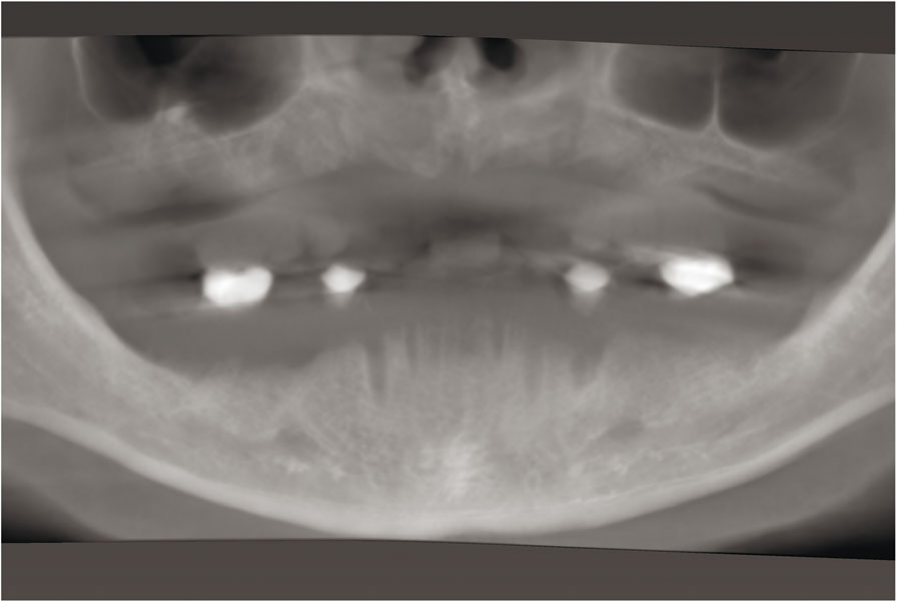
Pre-operative CBCT with radiographic template and radiopaque teeth
